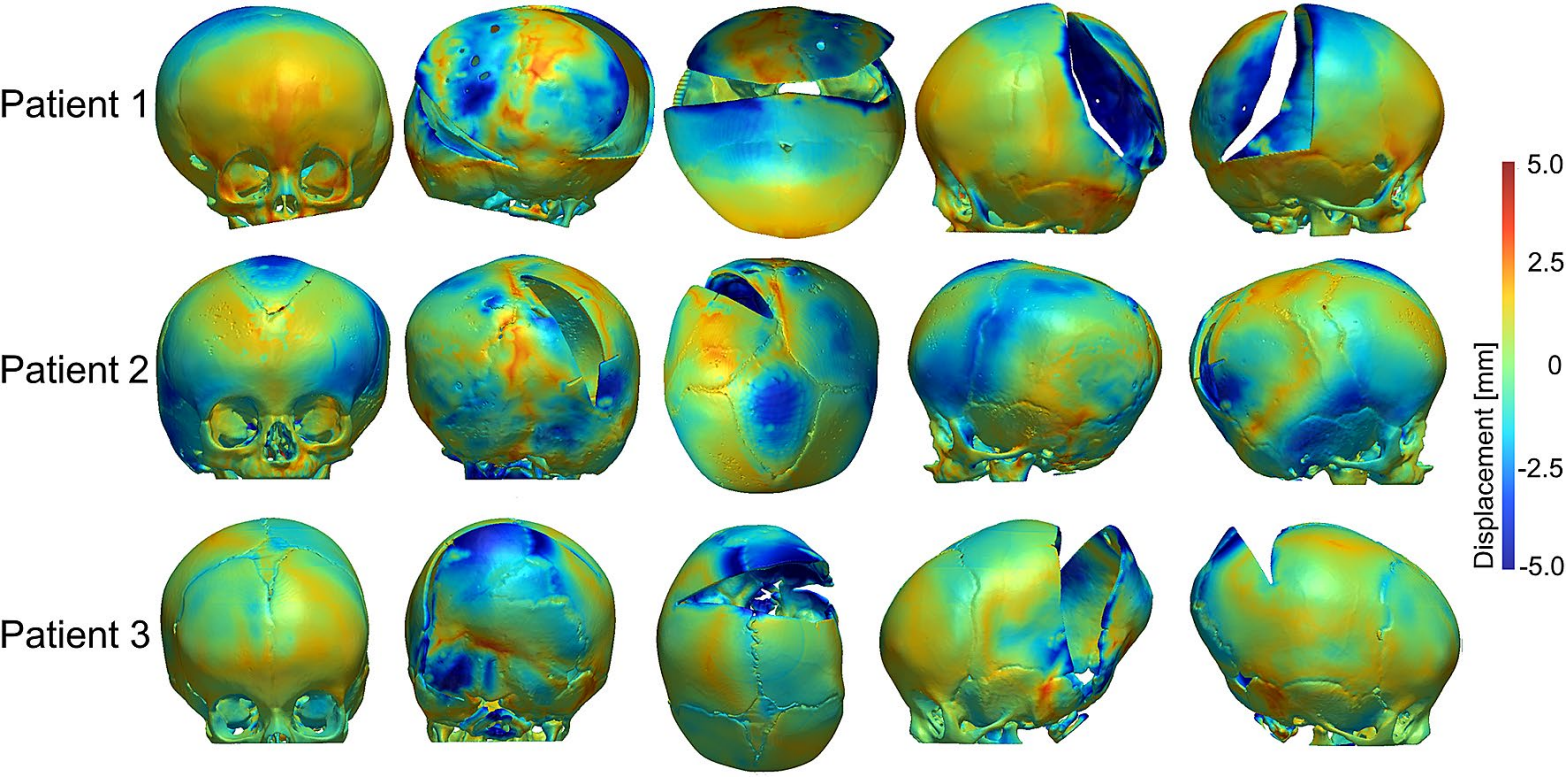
Surface deviation between the FE models and post-operative skull models reconstructed from CT images.
